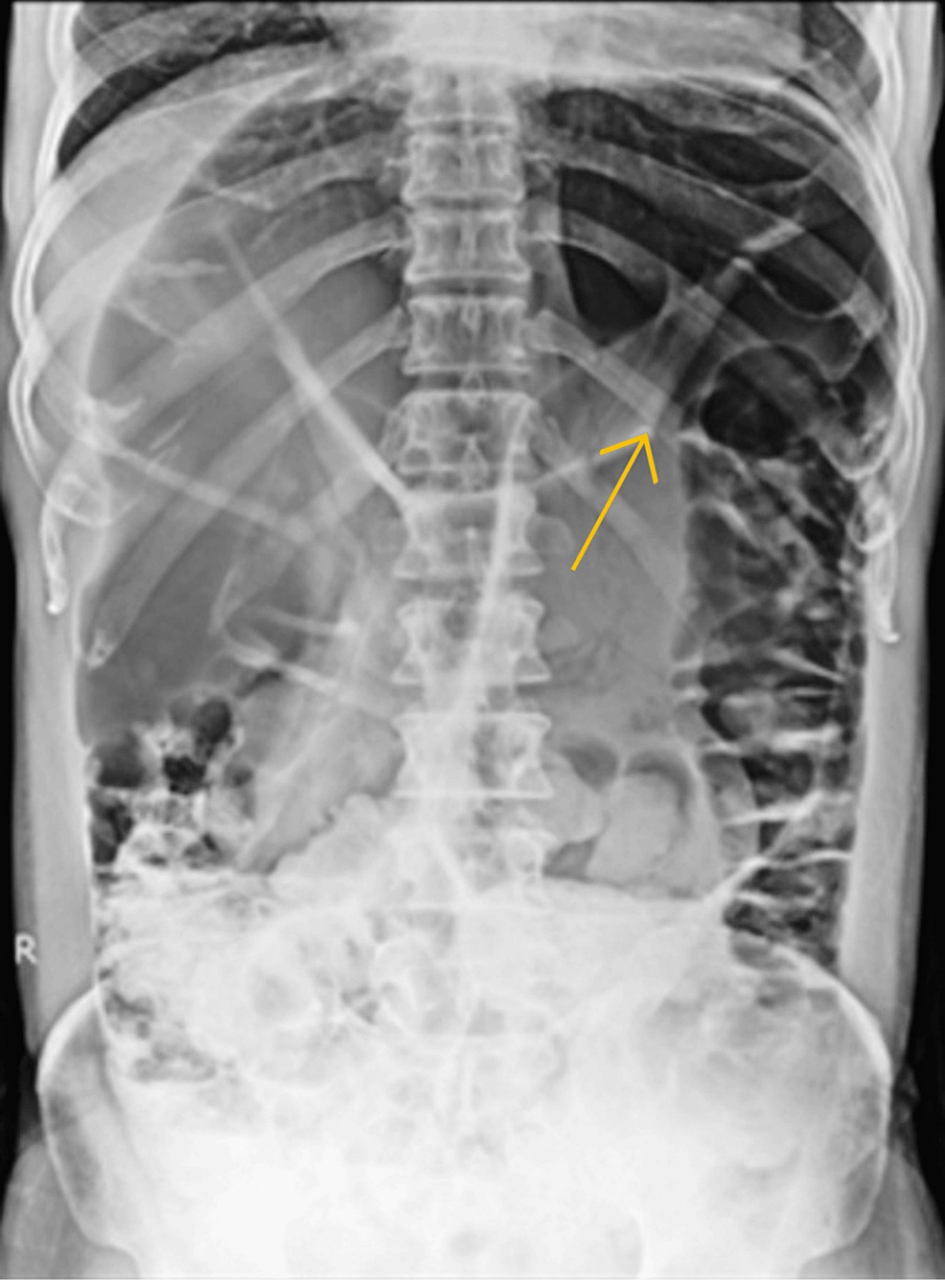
X-ray revealing a dilated sigmoid loop with a classic coffee bean sign The arrow shows the coffee bean sign.
